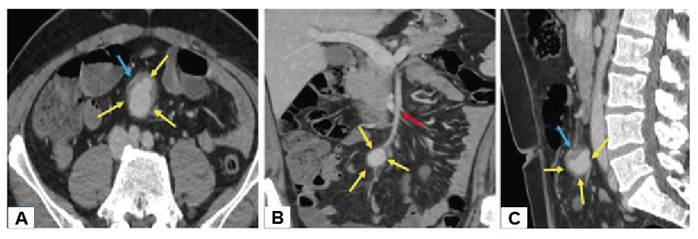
Axial (A), coronal (B), and sagittal (C) plane abdomen CT shows aneurysm (yellow arrows) of the superior mesenteric artery (red yellow) and perivascular fat stranding and inflammatory soft tissue (blue arrows).
